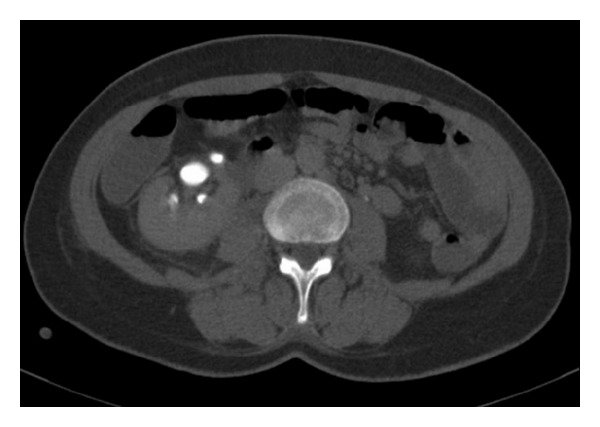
After treatment, no escape of contrast material from the pelvis renalis is seen at the delayed phase contrast enhanced control CT scan.
